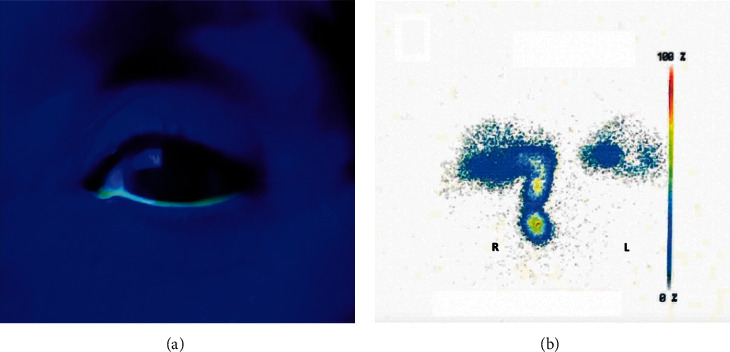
Delay of lacrimal transit on the left eye after 5 minutes demonstrated with fluorescein dye disappearance test (FDDT) (a) and dacryoscintigraphy (b).
